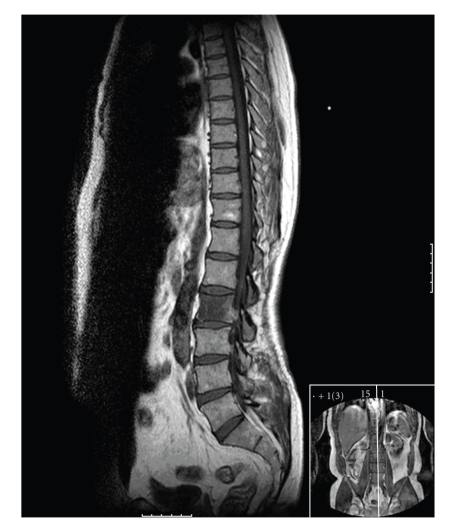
The MRI scan shows homogeneous isointense signal relative to skeletal muscle on T1-weighted images throughout L2 vertebra. The cortex is intact.
